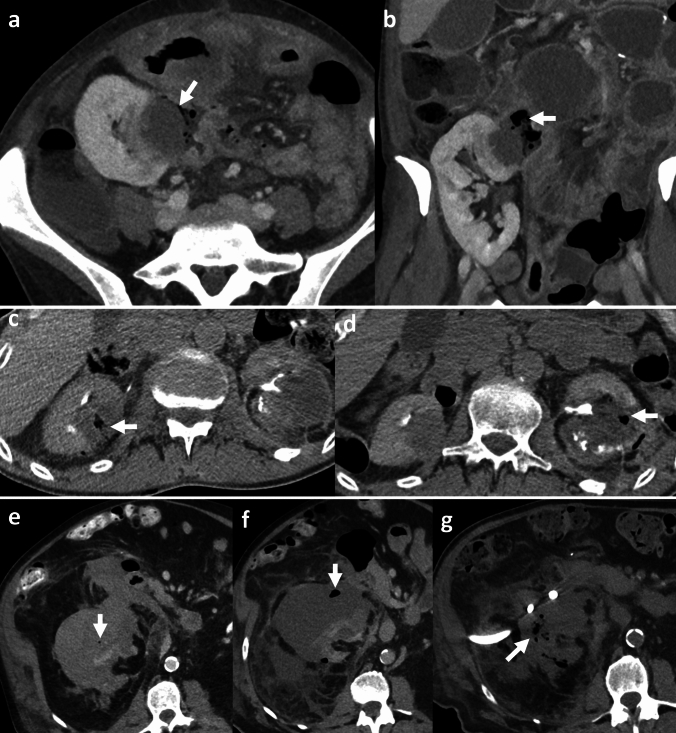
Pitfalls for emphysematous pyelonephritis. a, b Nephro-enteric fistula: A 35-year-old post renal transplant complicated by renal abscess and fistula with adjacent small bowel (white arrows, a, b). Air in the renal abscess is due to a bowel fistula. c, d Post-ablation changes. A 54-year-old with bilateral renal tumors treated with ablation on two different sessions. Post-procedure CT images show expected air foci in bilateral ablated regions (white arrows c, d), which resolved on follow-up. e–h Post-embolization changes with infection. A 71-year-old with end-stage renal disease and a spontaneous atraumatic right perirenal hematoma with active bleeding treated with right renal artery embolization. CT performed 3 days later shows (e) expected post-procedural small speck of air (white arrow, e). Follow-up CT at 6 weeks f shows worsening air foci and increased perinephric fat stranding suggestive of new emphysematous infection (white arrow, f). Follow-up CT at 3 months g after drain placement shows worsening air foci in the atrophied kidney (white arrow, g) and persistent perinephric inflammation. Right nephrectomy was thus performed. Drain contents showed *Pseudomonas aeruginosa*
